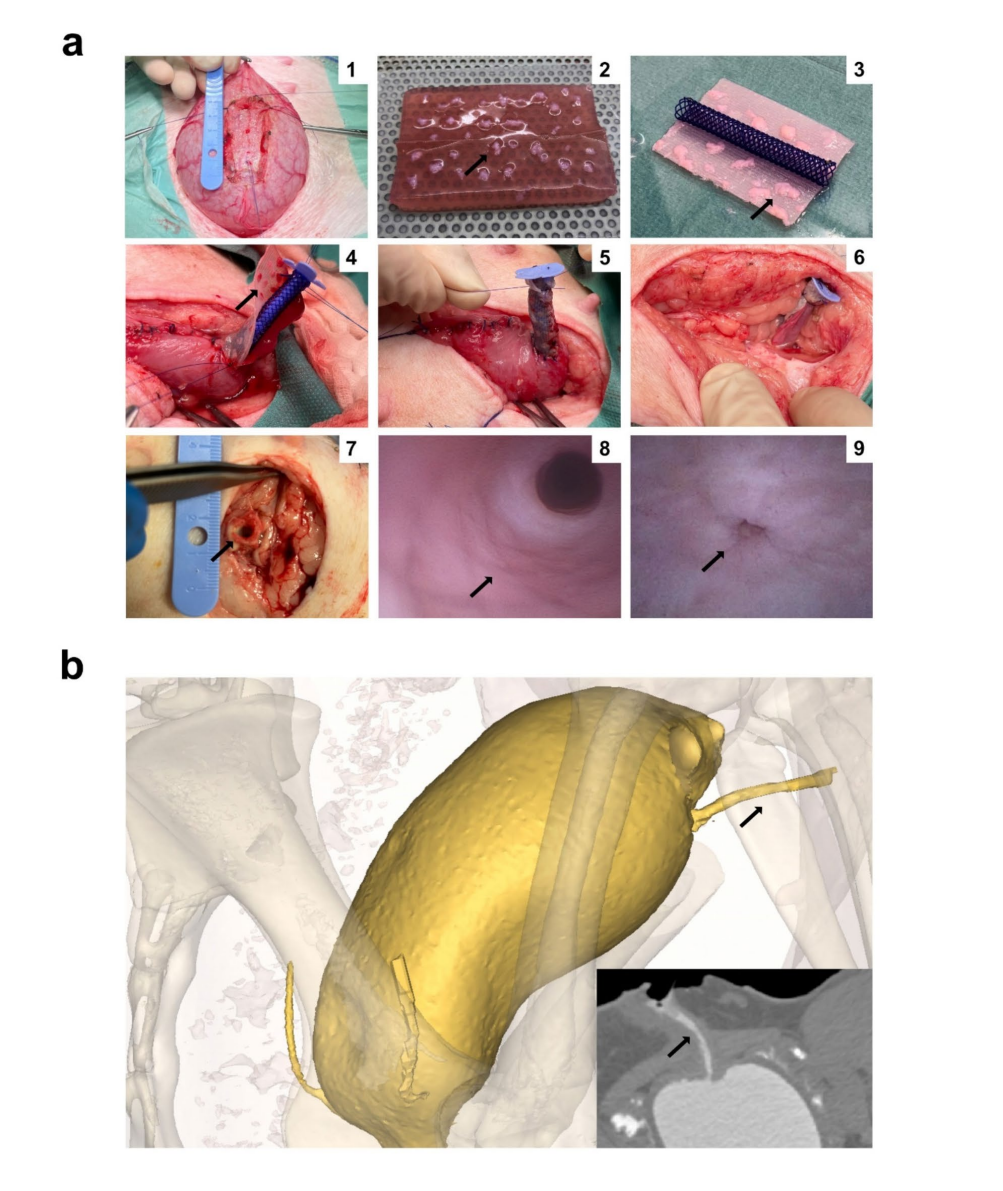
Perioperative scaffold construction and postoperative evaluations. (a) A segment of tissue was prepared for resection from the anterior bladder wall before further dissection and mincing into mucosal micrografts (1). The micrografts (marked) were embedded into the mesh-reinforced collagen (2). The compressed scaffold with adhescent micrografts (marked) before tubularization around the stent (3). Conduit implantation with luminally facing micrografts (marked) on the anterior bladder wall (4). Placement of the endoluminal ACE stopper and ligation of the distal conduit (5). Trans-fascial subcutaneous fixation of the ligated conduit (6). Post-mortem dissection of the conduit with clear urine in the lumen (marked) (7). Visible stent remnants (marked) seen with cystoscopy via the external conduit opening (8). Internal bladder anastomosis (marked) seen with cystoscopy via the native urethra (9). (b) Two- and three-dimensional representations of the bladder and conduit (marked) from post-mortem CT urography.
